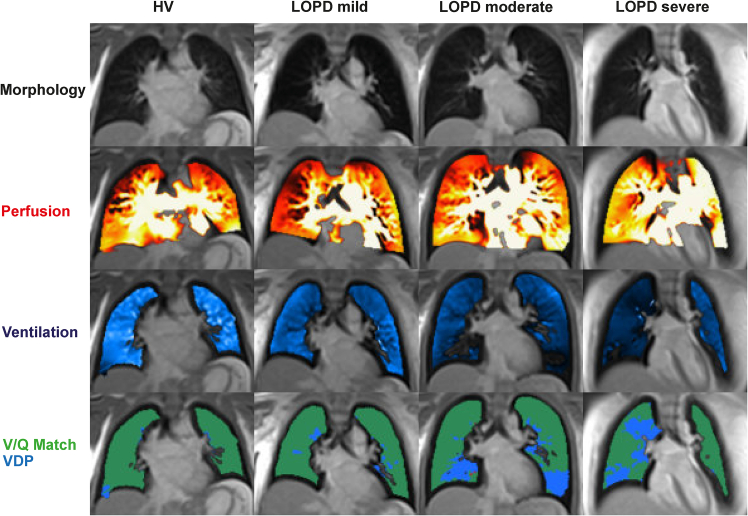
Representative PREFUL MRI images based on disease severity Free-breathing phase-resolved functional lung (PREFUL) low-field-strength magnetic resonance imaging (MRI) at 0.55 T, with calculated parameters in the axial plane after automatic registration to a mid-expiration position and lung parenchyma segmentation. From left to right, representative MRI lung morphology of a healthy volunteer (HV) and a LOPD patient of increasing disease severity according to their vital capacity (VC). We classified patients with a normal VC of 100%–88% as LOPD mild, patients with a VC of 87%–58% as LOPD moderate, and patients with a VC of 57%–0% as LOPD severe. From top to bottom, representative color-coded images from functional lung MRI show perfusion, ventilation, ventilation defects (blue), and ventilation-perfusion (V/Q) match (green). VDP, ventilation defect percentage.
